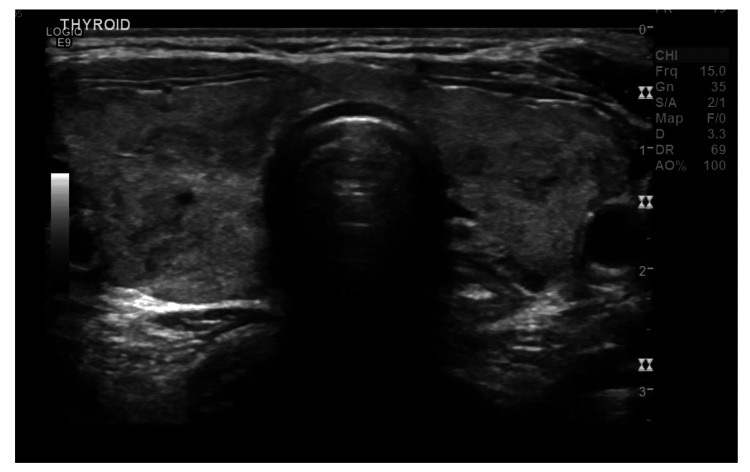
Thyroid ultrasonography demonstrated an enlarged heterogeneous gland and bilateral low-risk nodules without suspicious cervical lymphadenopathy.
